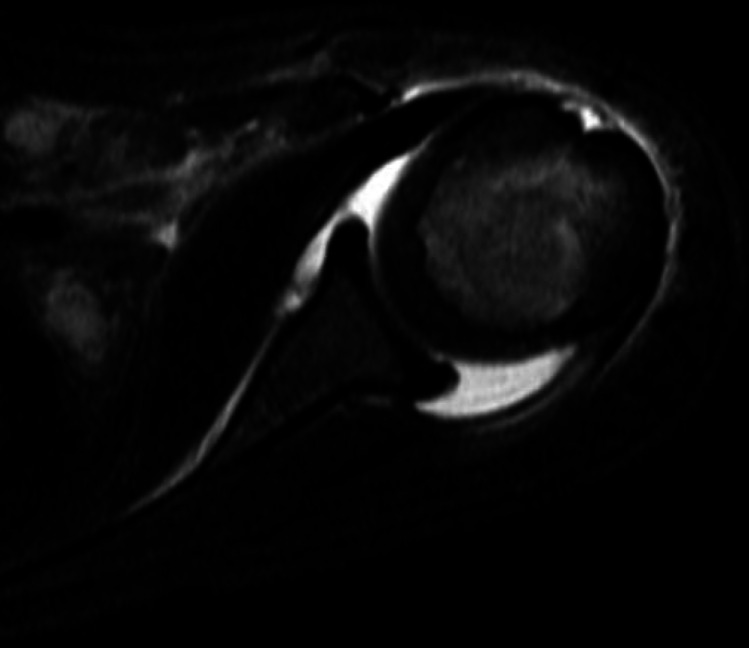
MRI of a shoulder showing joint effusion in setting of a septic joint
